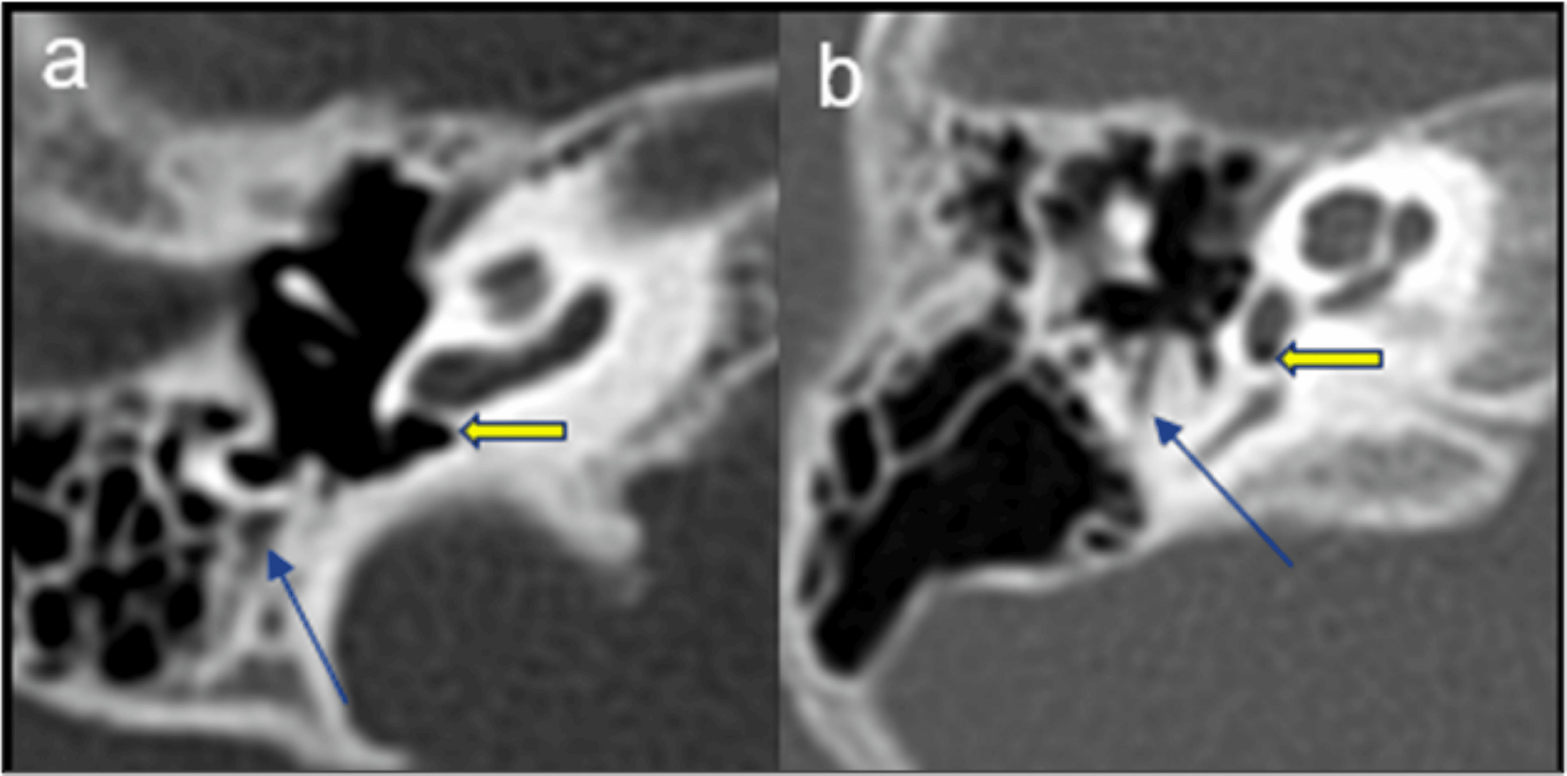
(a) and (b) Axial images of HRCT temporal bone demonstrating descending segment of the facial nerve canal. (a) Normal and (b) overhanging the round window. Blue arrow: descending segment of facial nerve, yellow arrow: round window. HRCT: high-resolution computed tomography.
